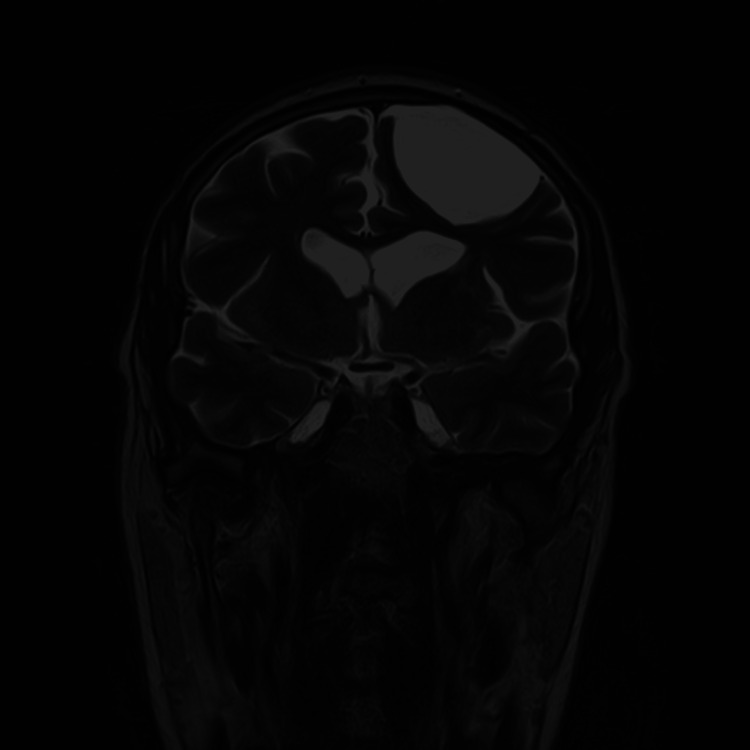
Magnetic Resonance Imaging showing a large left frontal arachnoid cyst - Coronal view.
